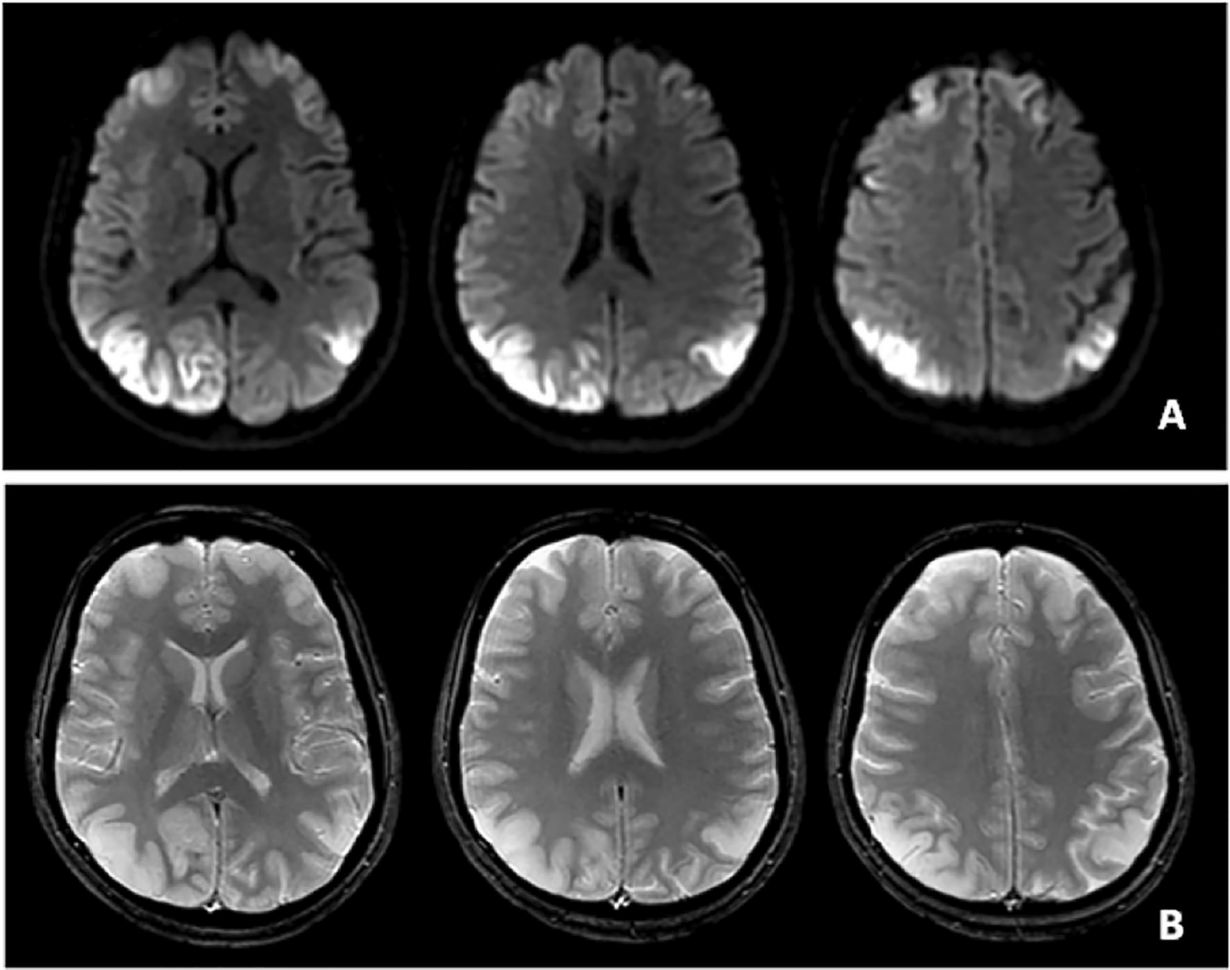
DWI-MRI (Panel A) and T2-weighted images (Panel B) of a patient with molecular diagnosis of mitochondrial disease. DWI hyperintensities in the occipito-parietal cortex may be suggestive of CJD, but findings of cortical swelling in the corresponding regions in T2-weighted images did not confirm the suspect of prion disease
